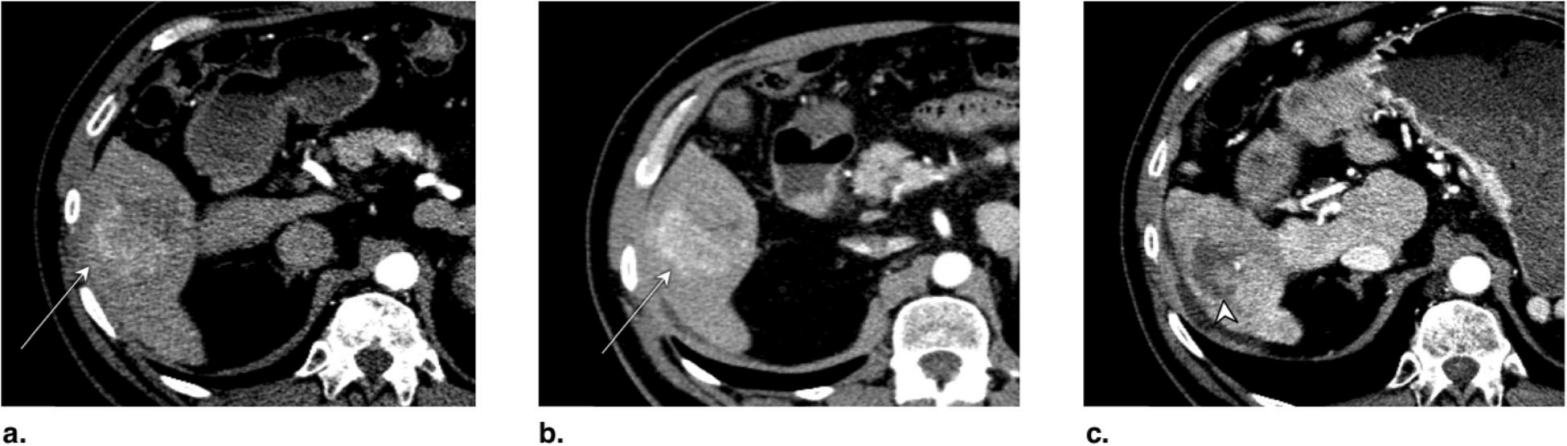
Axial CT in late arterial phase obtained (a) pretreatment: image shows an enhancing 6-cm LR-5 observation in segment VI (arrows). b 1 month post transarterial radioembolization (TARE): image shows no change. c 6 months post TARE: image shows atrophy and diffuse enhancement of segment VI, hypoenhancement at the anterior aspect of the lesion and faint posterior enhancement (arrowhead). These changes may represent treatment-specific expected enhancement pattern or residual tumor. The treated observation is categorized LR-TR Equivocal
